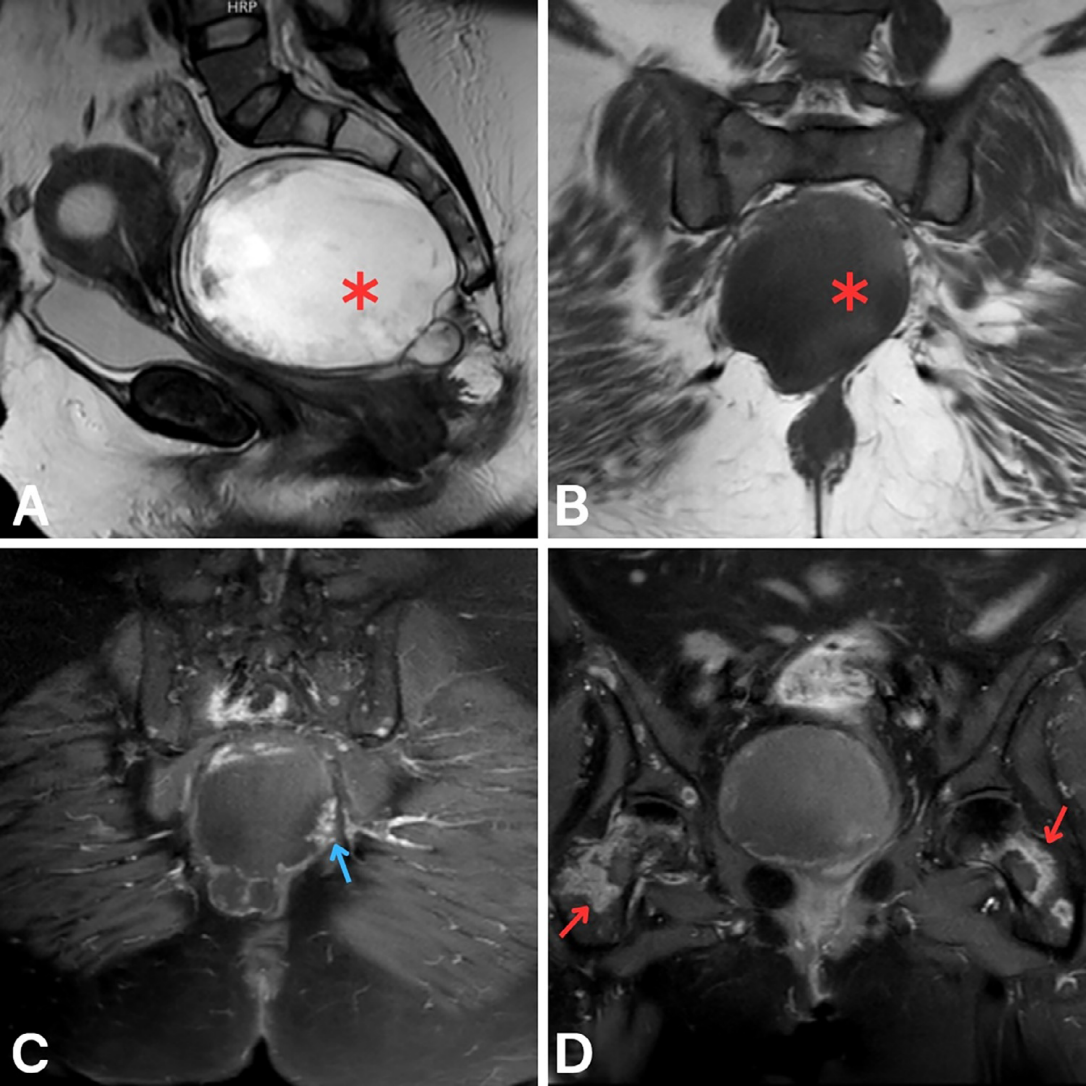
Follow-up pelvic MRI with and without intravenous gadolinium contrast. (A) Sagittal T2-weighted image showing a hyperintense presacral lesion with a well-defined wall (red asterisk). (B) Coronal T1-weighted image (precontrast) showing the presacral lesion as hypointense (red asterisk). (C,D) Coronal T1 fat-saturated images postcontrast showing peripheral nodular enhancement (blue arrow) and enhancing bony metastases (red arrows).
